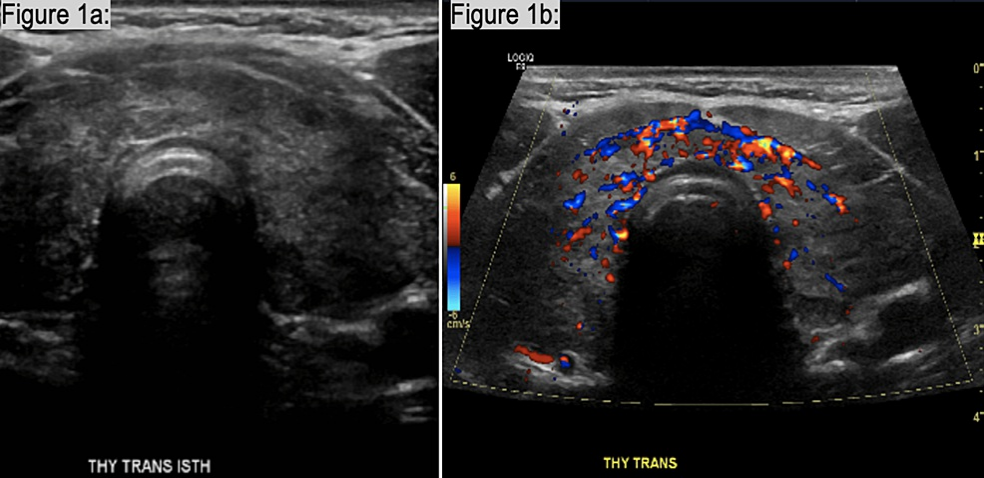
Thyroid transverse views of the isthmus. (a) Heterogenous regions of thyroid with no apparent lesions. (b) Color Doppler showing increased vascularity in the thyroid.
